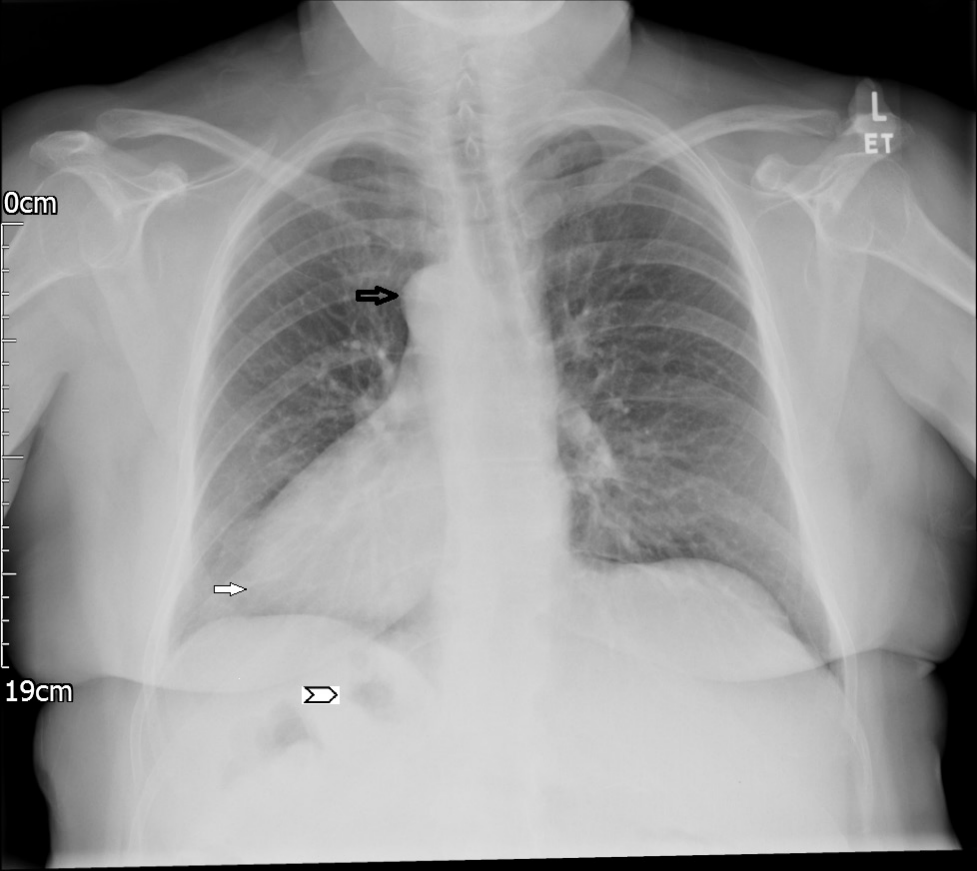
Frontal chest radiograph showing the aortic knuckle (black arrow), apex of the heart (solid white arrow) and gastric bubble (arrowhead) all on the right.
